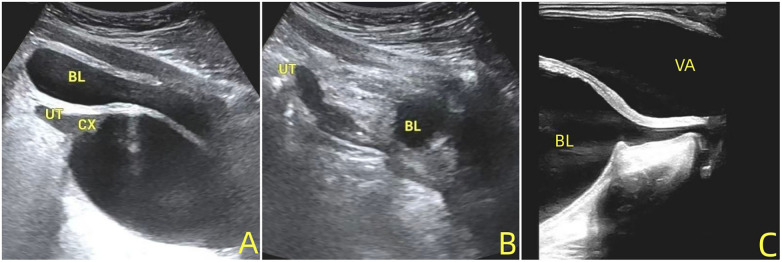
(A): transabdominal ultrasound shows a large anechoic fluid collection distending the vagina before voiding; (B): transabdominal ultrasound shows disappearance of the anechoic fluid collection in the vagina after voiding; (C): transrectal ultrasound shows a large anechoic fluid collection distending the vagina before voiding. BL, Bladder; UT, Uterus; VA, Vagina.
